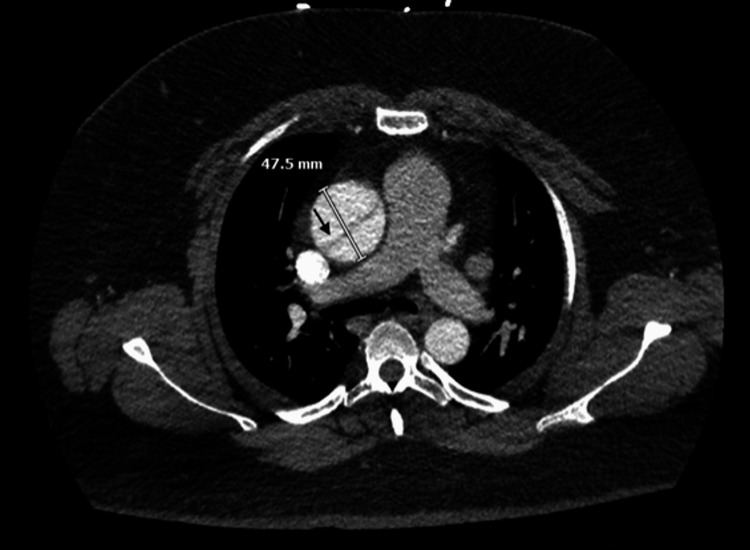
Chest computed tomography angiography showing aortic root dilation and aortic dissection flap as highlighted by the black arrow
